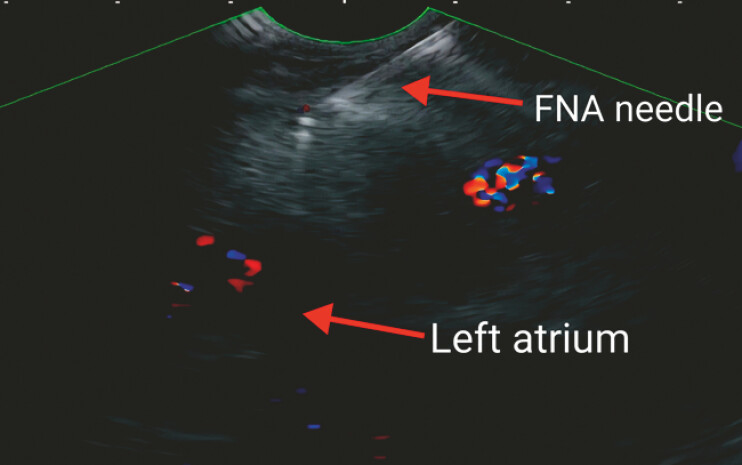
Puncture of the left atrium was performed under EUS guidance. EUS, endoscopic ultrasound.
